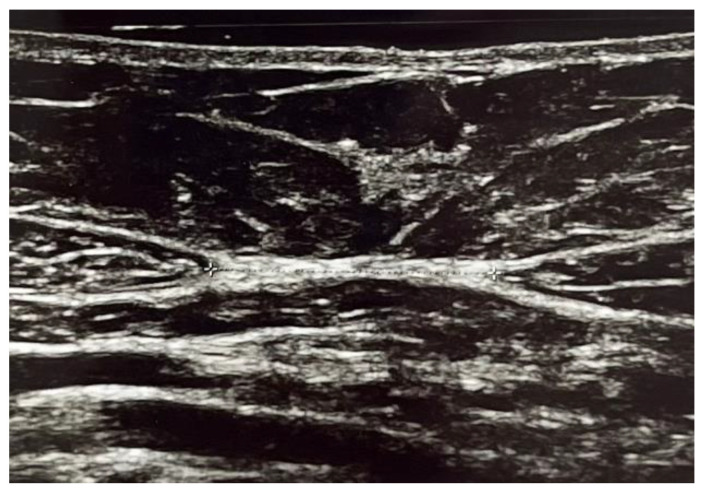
Ultrasound image of supraumbilical inter-rectal space.
